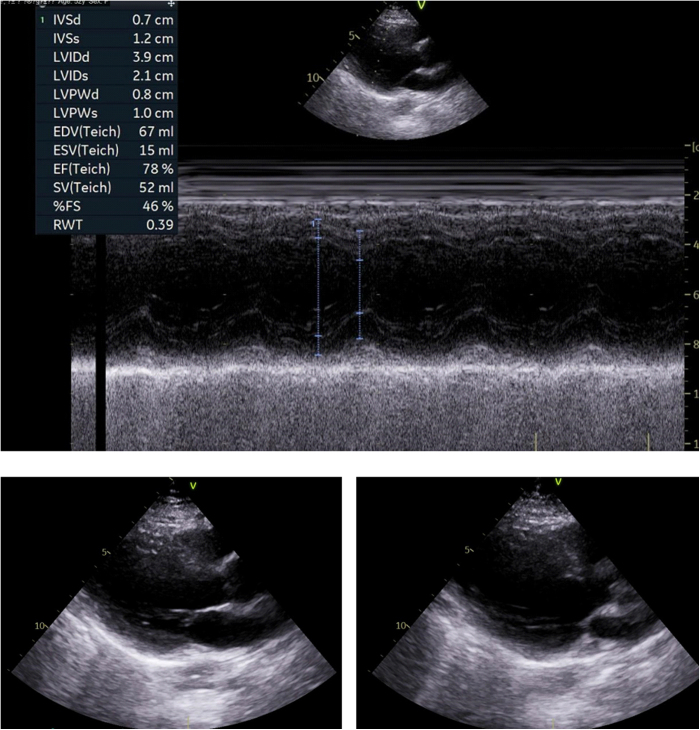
Echocardiogram results of the patient.
